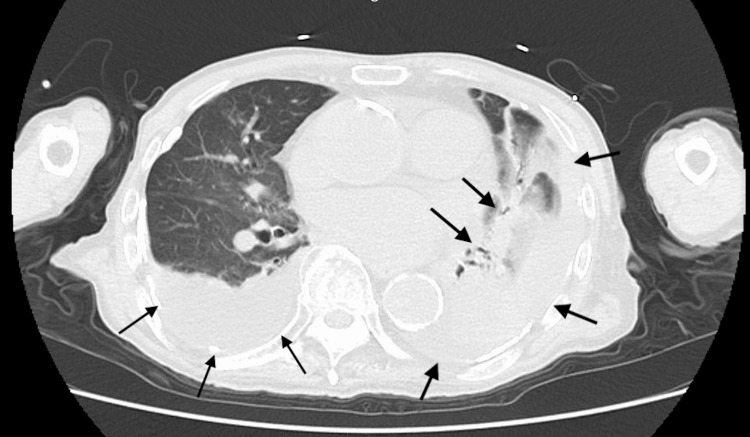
Chest CT images on arrival at the emergency department showing bilateral pleural effusions and infiltrative shadows predominantly in the left lung (black arrows).
